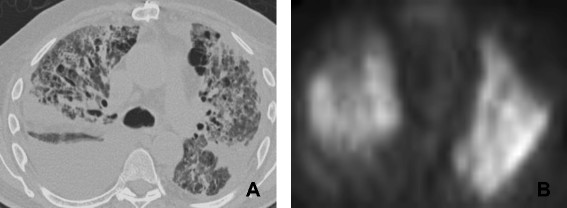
66 year old male with post transplant lymphoproliferative disorder on rituximab. A. CT scan of the chest shows diffuse abnormality with bilateral effusions, ground glass attenuation, thickened interstitium and coarse linear parenchymal bands. B. PET image shows diffuse parenchymal uptake of FDG in keeping with a diffuse inflammatory process. Biopsy revealed diffuse alveolar damage.
